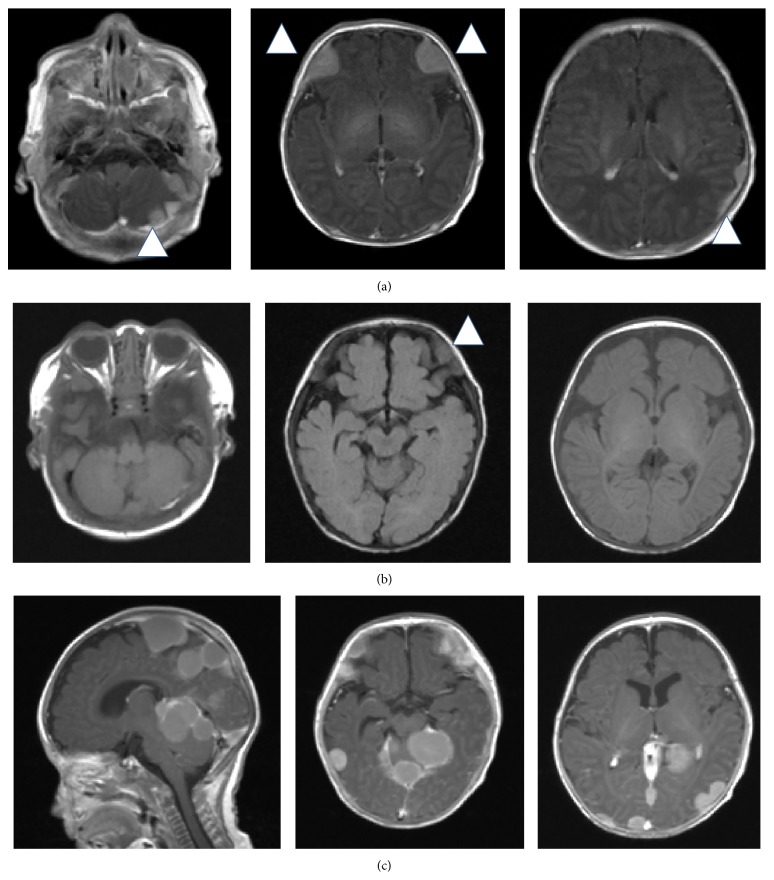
Magnetic resonance imaging studies of the brain: (a) a gadolinium-enhanced T1-weighted image at 7 days of age, (b) a T1-weighted image at 45 days of age, and (c) a gadolinium-enhanced T1-weighted image at 72 days of age.
